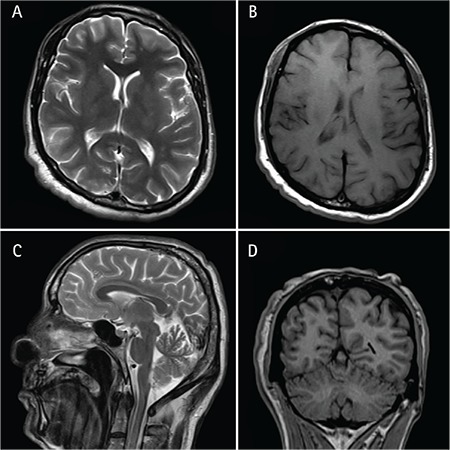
Magnetic resonance imaging of patient V:6 in axial (A and B), sagittal (C), and coronal (D) planes.
